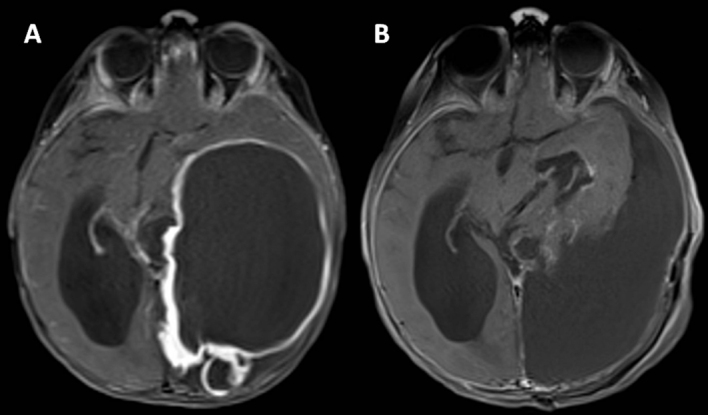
A. Brain MRI (post-contrast axial T1-weighted) revealed a
